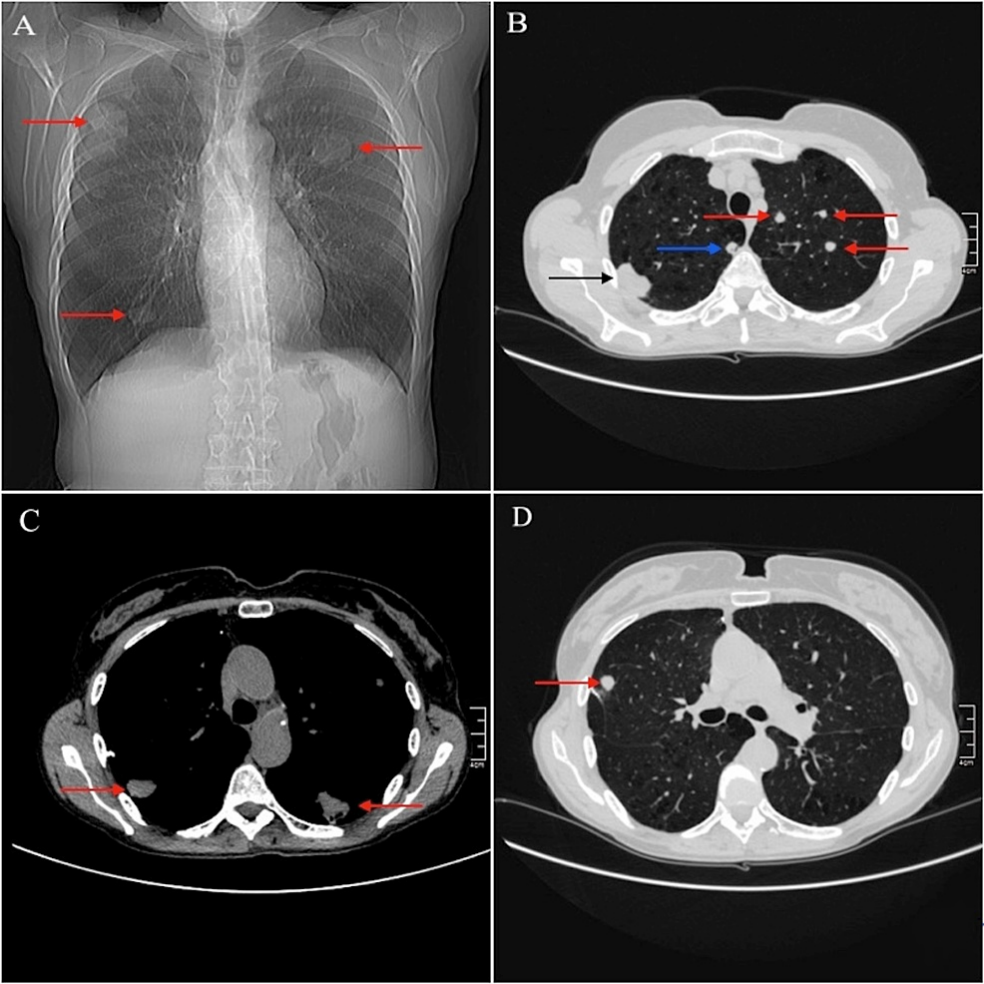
Chest CT showing multiple pulmonary nodules (A) A chest CT scout image showing multiple bilateral nodules within the lung parenchyma, indicated by red arrows; (B, D) CT images in lung window settings. A prominent right-sided lesion measuring 25 x 50 x 50 mm, located in the S2 pulmonary segment, is highlighted by a black arrow. A small right-sided nodule in the S2 pulmonary segment, in contact with the mediastinal pleura, is indicated by a blue arrow. Additional smaller bilateral nodules are depicted by red arrows. (C) A CT image in mediastinal (soft tissue) window setting. Two nodules in direct contact with the parietal pleura are marked by red arrows. The left-sided lesion exhibits partial cavitation.
